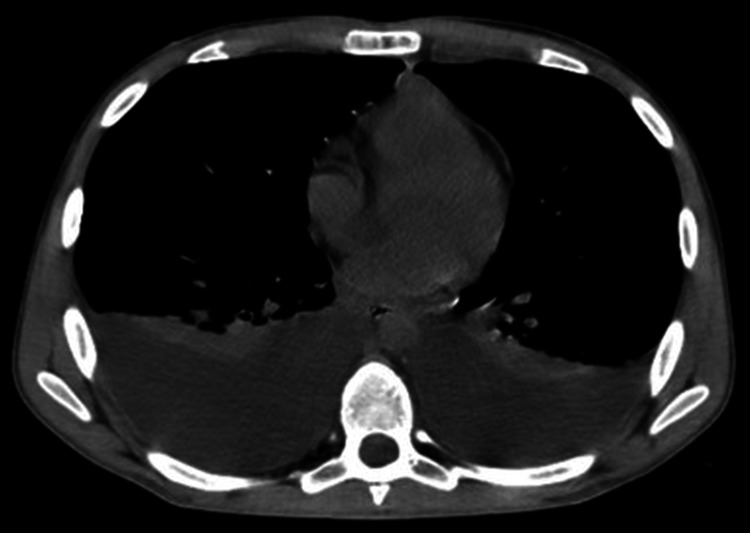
Thoracic CT: bilateral pleural effusion. CT: computed tomography
